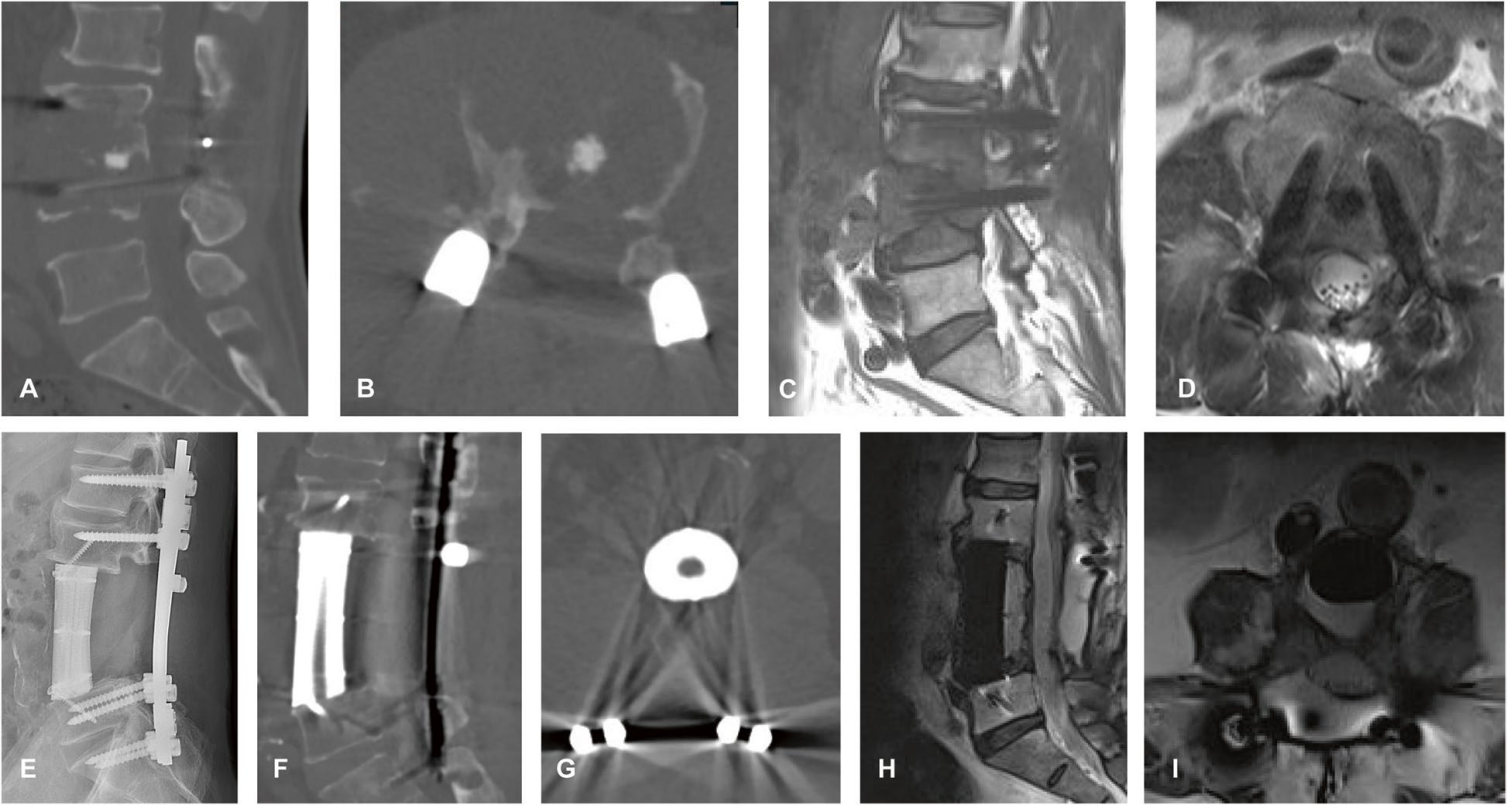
A 59-year-old man with a medical history of surgery for disc herniation complained of increasing back pain during the previous 1 month, his KPS score was 60, and the American Spinal Injury Association impairment scale was D. The patient underwent TES and spine reconstruction with customized 3D-printed AVB via a one-stage combined approach, and the estimated blood loss was 2400 mL. Intraoperatively, inferior vena cava injury was developed and repaired. A, B Preoperative CT scan revealed osteolytic body destruction in L3 and L4 vertebral. C, D Preoperative sagittal and axial T2-weighted MRI. E Postoperative lateral radiograph. F, G Postoperative CT scan showing an excellent position of the 3D-printed AVB. H, I, MRI scan at a 12-month follow-up showing no tumor recurrence. Abbreviations: KPS, Karnofsky performance status; TES, total en bloc spondylectomy; 3D, three-dimensional; AVB, artificial vertebral body; CT, computed tomography; MRI, magnetic resonance imaging
